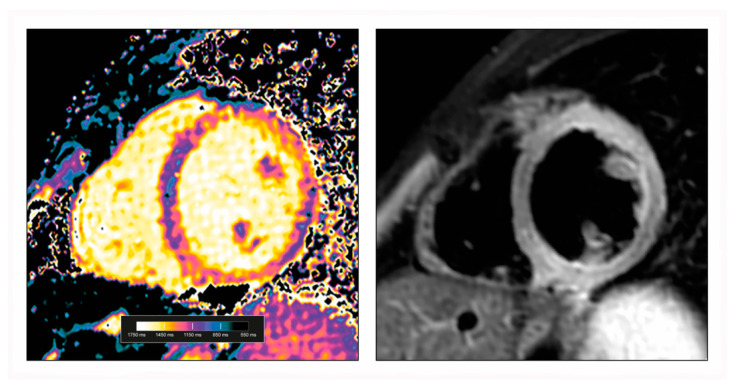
Native T1 map (left) in a patient with acute myocarditis showing prolonged T1 relaxation times in the anterolateral and inferoseptal regions of the left ventricle (1320 ± 43 ms (local reference 999 ± 31 ms)). Corresponding T2-weighted black blood short tau inversion recovery sequence (right) with clear signs of oedema in the same regions.
